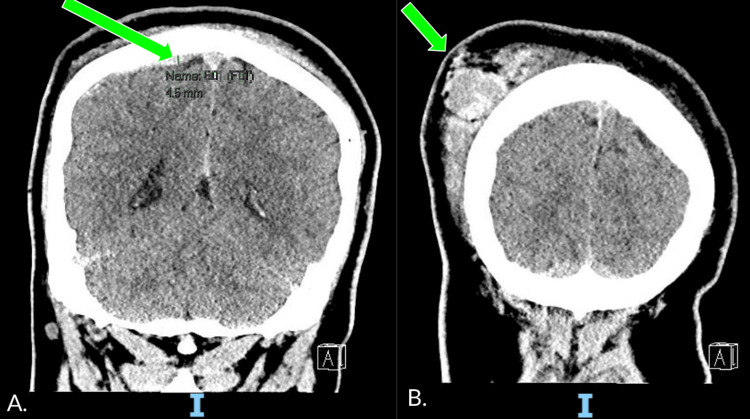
CT head without IV contrast performed on admission A. CT head showed a thin, acute high right parietal convexity subdural hematoma measuring up to 4-5 mm in maximum thickness, pointed out by the green arrow. There is no significant mass effect. B. Large right parieto-occipital scalp hematoma, pointed out by the green arrow.
